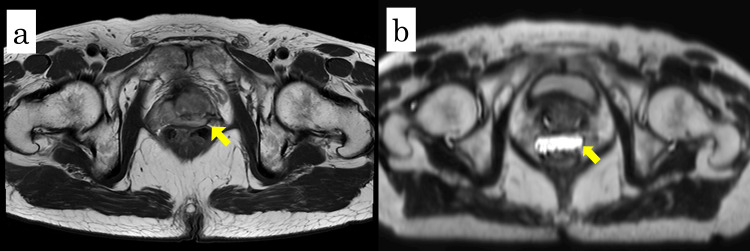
Pre- and post-spacer MRI findings (a) T2-weighted axial magnetic resonance imaging (MRI) before spacer placement showing close approximation between the prostate and rectum (yellow arrow). (b) T2-weighted axial MRI after spacer placement demonstrating a hyperintense area (yellow arrow) corresponding to the hydrogel spacer, indicating adequate separation.
